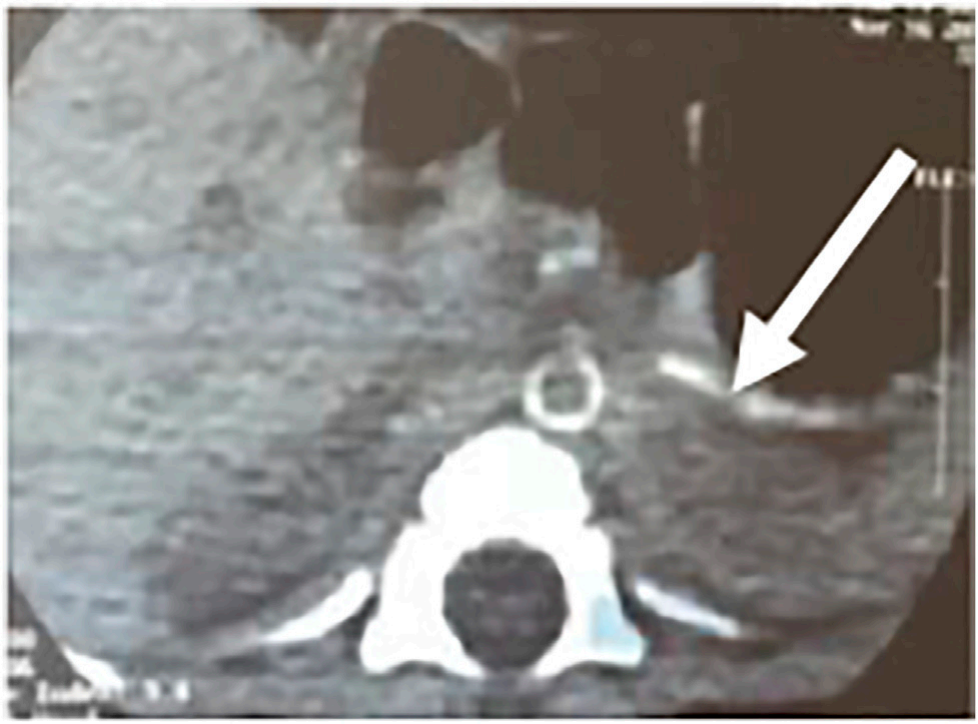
Enhanced contrast CT scan of the abdomen shows calcification of the abdominal aorta and its branches.
